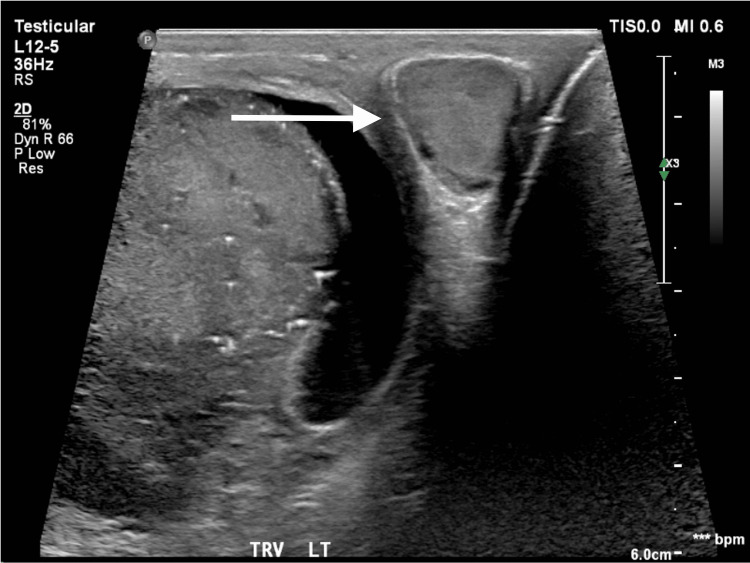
Ultrasound of the scrotum demonstrating a homogenous mass
